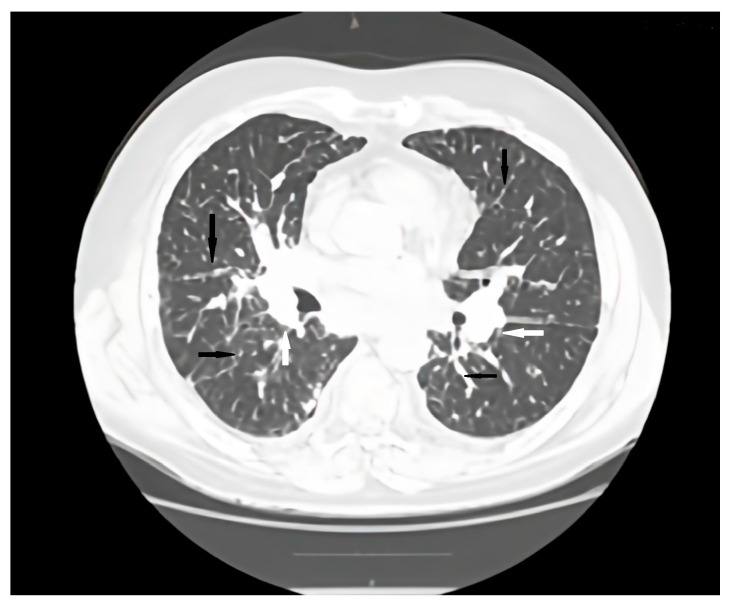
Axial CT scan pulmonary window evaluation after 6 months. A, anterior; P, posterior; black arrows, irregular peribronchovascular thickening; white arrows, adenopathy.
